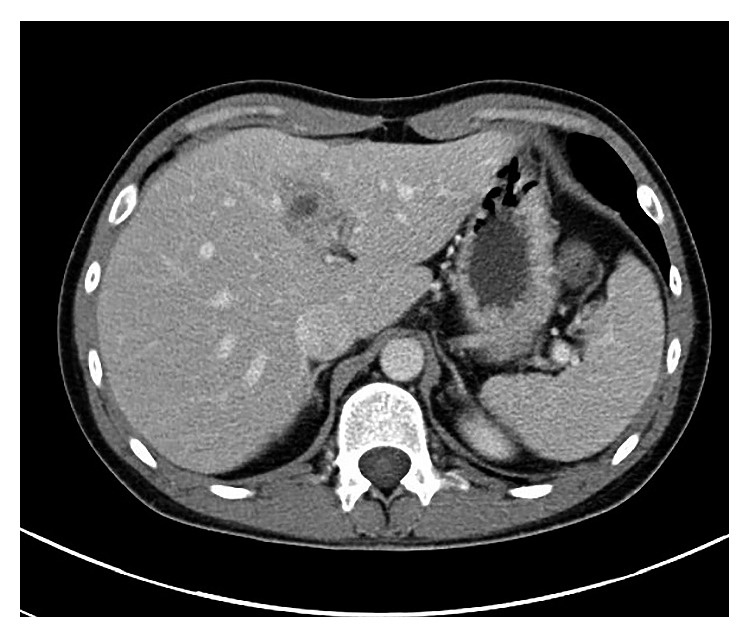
Abdominal CT scan 2 weeks after discharge demonstrating near total resolution of the previously seen hepatic segment 4 abscess without any drainable fluid collection.
